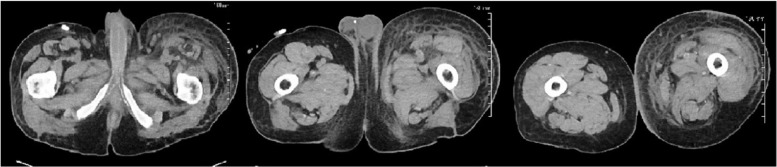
Computed tomography scan of pelvis without contrast showed extensive edema and congestion of the subcutaneous fat extending from the left external oblique musculature inferiorly along the lateral aspect of the pelvis into the upper left lower extremity.
